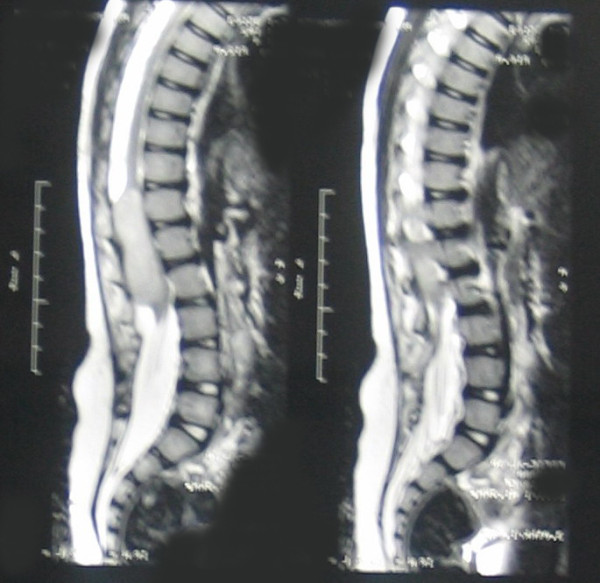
Sagittal T2-weighted MRI scan showing an isointense mass in the thoracolumbar area.
